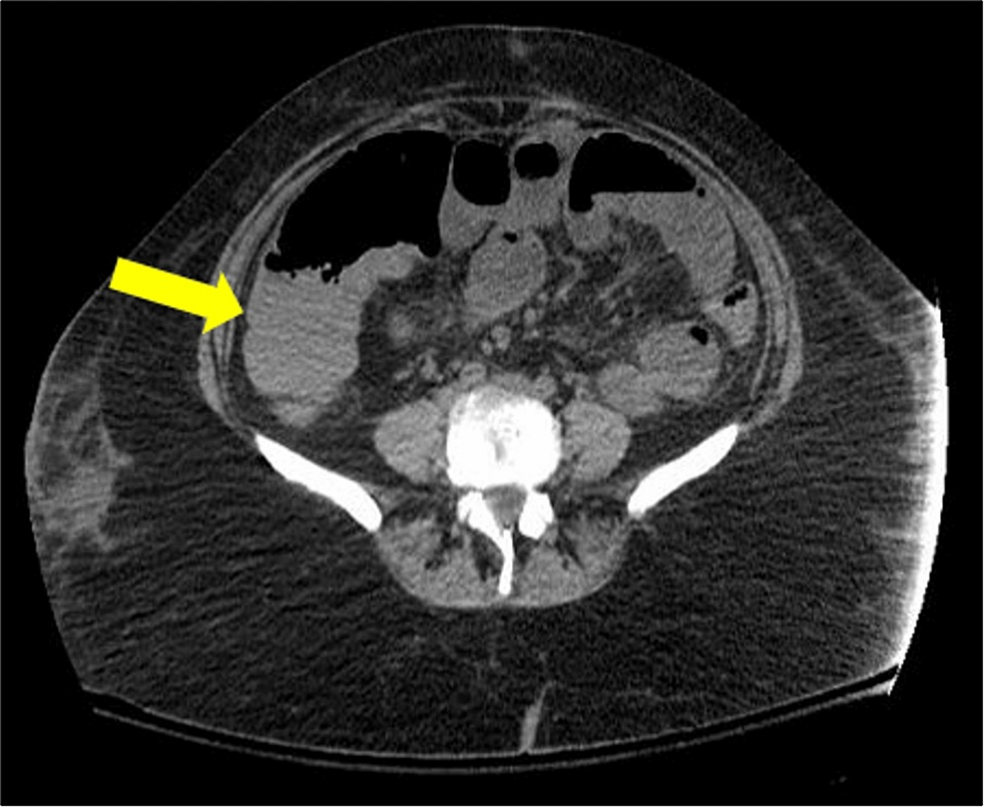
Posttreatment follow-up abdominal CT scan (axial view) that demonstrates resolution of pneumatosis coli with persistent distended bowel loops (yellow arrow). CT: computed tomography
